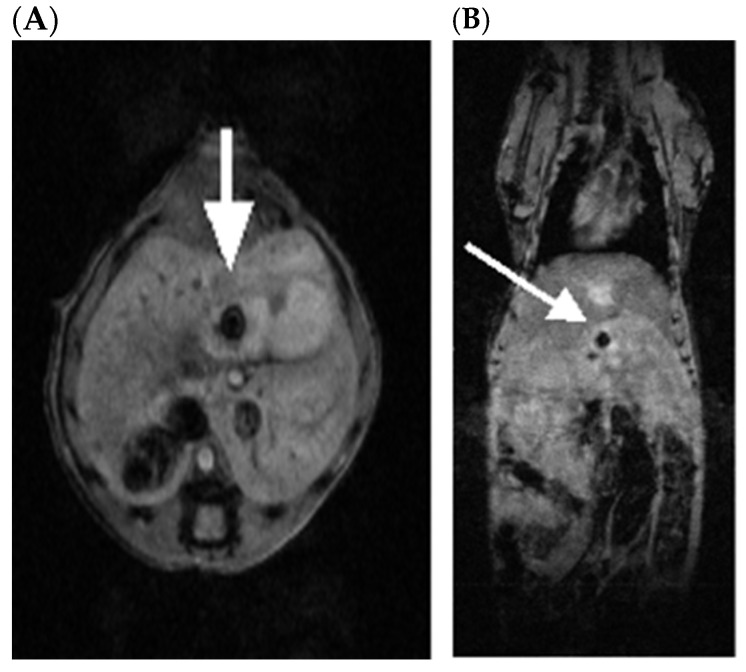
(A) Axial and (B) coronal images reveal low signal focus (arrows) in the pancreatic neck suspicious for tumor on day 21 after tumor cell inoculation. Imaging was performed using a gradient recalled echo (GRE) T1-weighted sequence. Following pilot scans to check alignment, T1-weighted GRE images were obtained in both the axial and coronal planes. Sequence parameters were as follows: TR 350 ms; TE 6 ms; NAV 8; Matrix 512 × 256 × 13 (coronal), 256 × 256 × 17 (axial); FOV 50 × 25 mm (coronal), 25 × 25 mm (axial); FA 65 deg. Spatial resolution was 98 × 98 × 750 µm.
